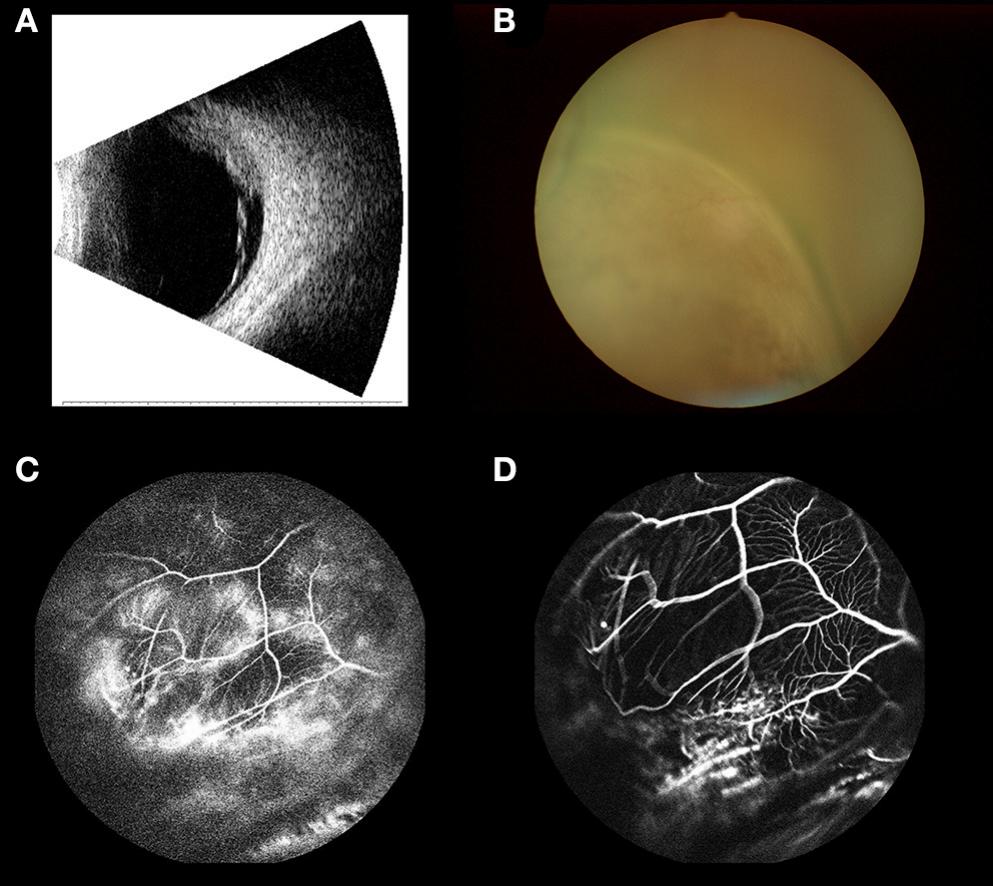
Ophthalmic imaging (ocular B-ultrasound, fundus photography, ICGA FFA) in patients with lung cancer ocular metastasis. (A) Ocular B-ultrasound. (B) Fundus photography. (C) ICGA. (D) FFA. ICGA, indocyanine green angiography; FFA, fundus fluorescein angiography.
